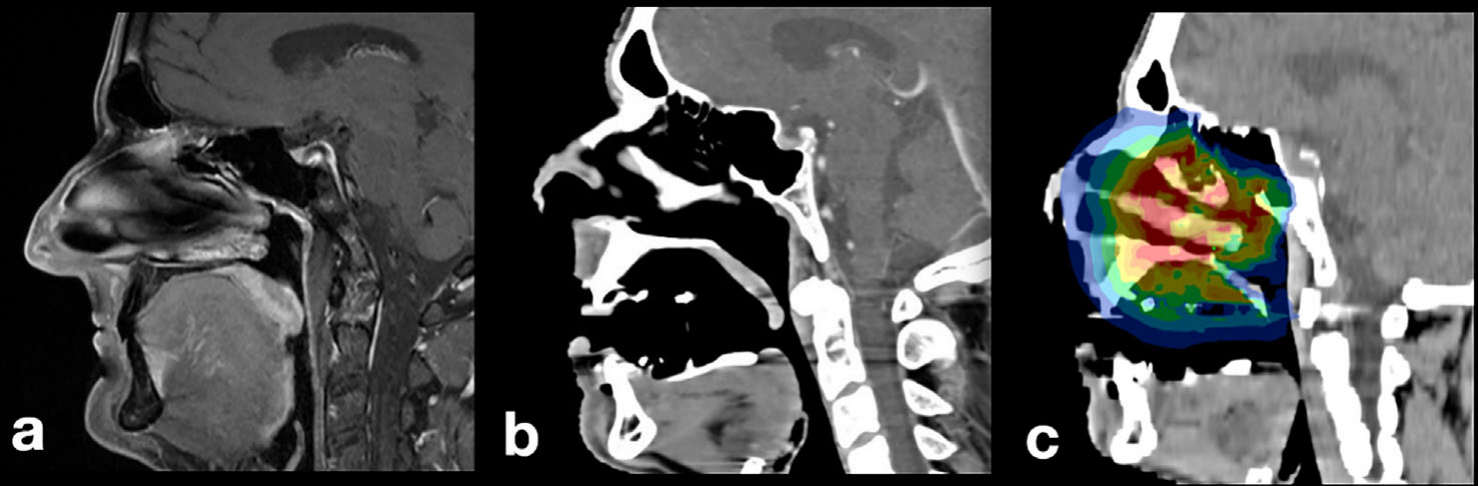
Patient with pleomorphic sarcoma of the nasal sinus: (A) diagnostic MR imaging without TRD, (B) baseline planning CT with incorporated TRD: the tongue is displaced to a caudal position, (C) irradiation plan without involvement of mandibular soft or hard tissues.
